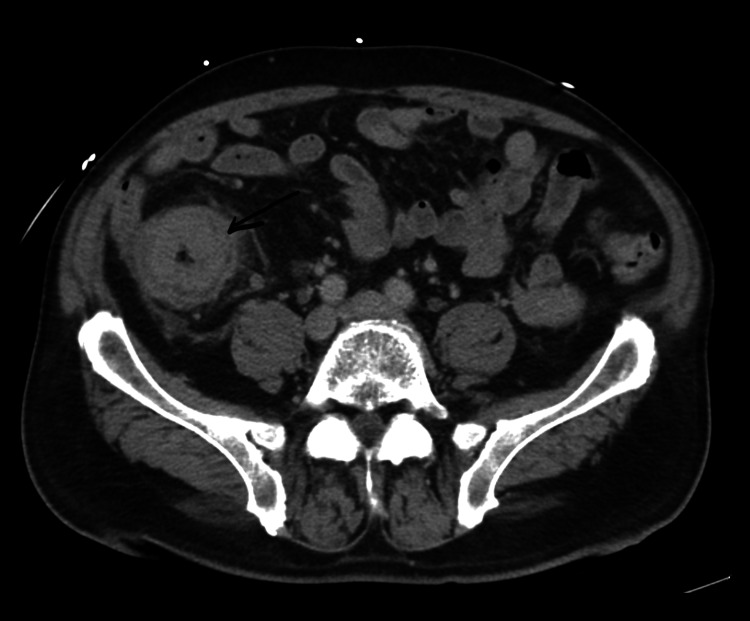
Contrast-enhanced CT of the lower abdomen shows circumferential wall thickening of the colon (black arrow), suspicious for primary site of colon cancer.
